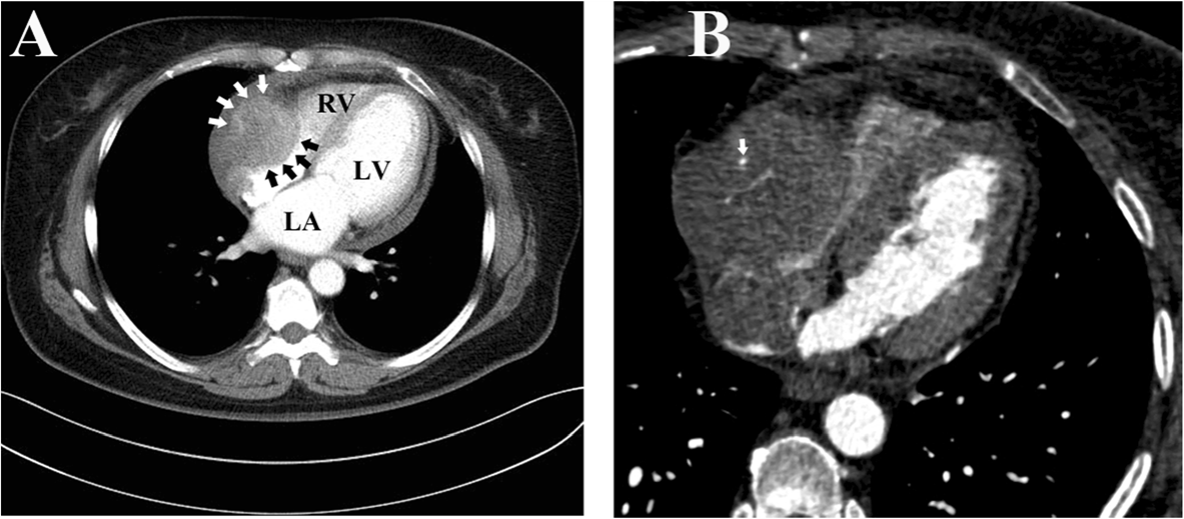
a. Computed tomography showing a tumour mass in the right atrium with irregular enhancement and, also a small pericardial effusion (arrow indicates the tumour). b. Computed tomography showing the right coronary artery was also involved by the tumour (arrow indicates the coronary artery)
